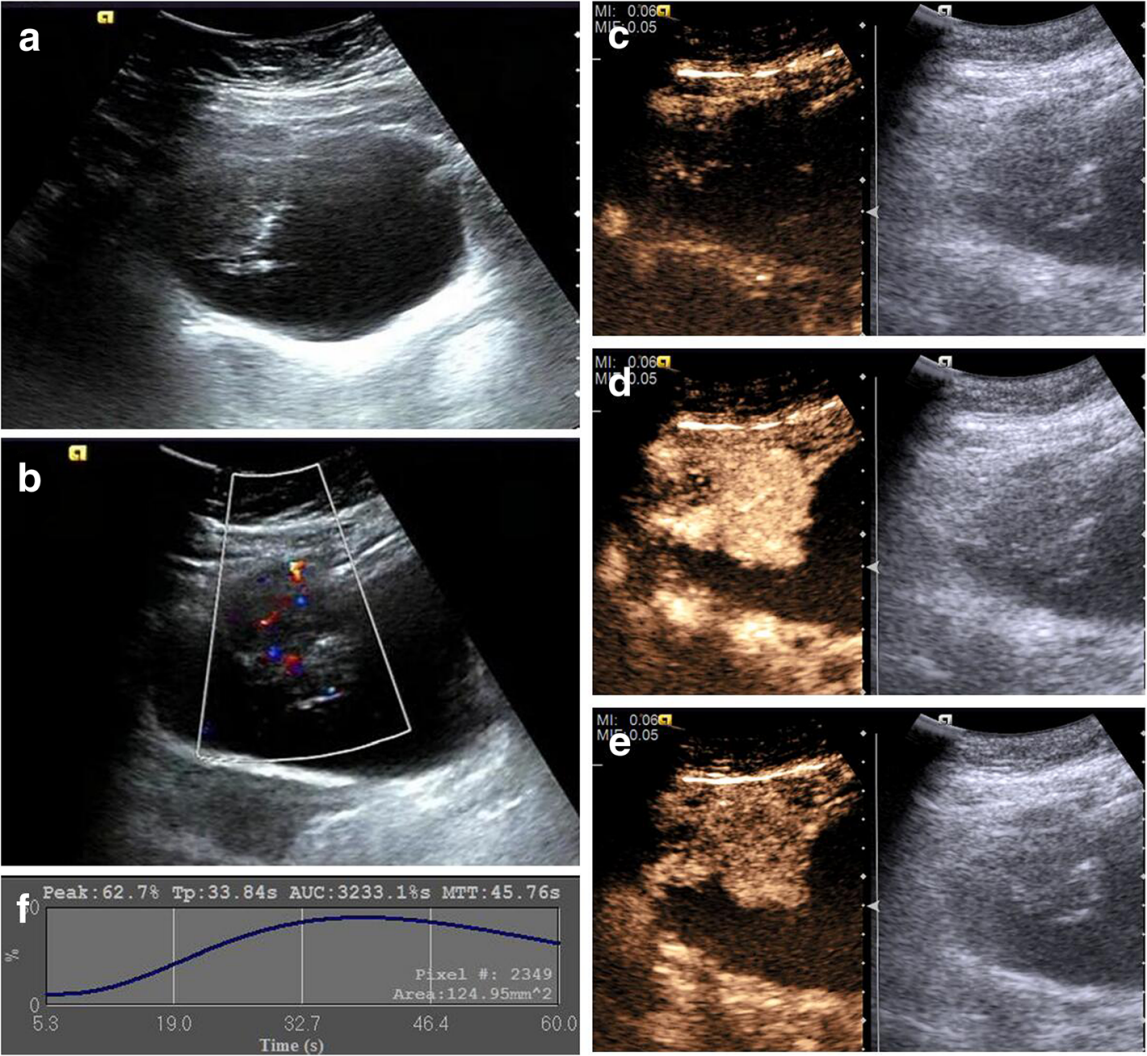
US images of small cell carcinoma of the bladder. a. Abdominal sonography revealed a hypoechoic mass in the apex of the urinary bladder. b. CDFI showed a rich blood flow signal inside this mass. c. CEUS imaging showed the mass began to undergo enhancement (wash-in time) from its periphery to its center at 10 s. d. CEUS imaging showed persistent high peak enhancement of the mass at 40 s. e. CEUS showed the enhancement signal of the mass was equal to the bladder wall at 120 s. f. Time-intensity curve showed wash-in time of 10 s, TTP of 33 s, SI of 62.7% and wash-out time > 60 s
